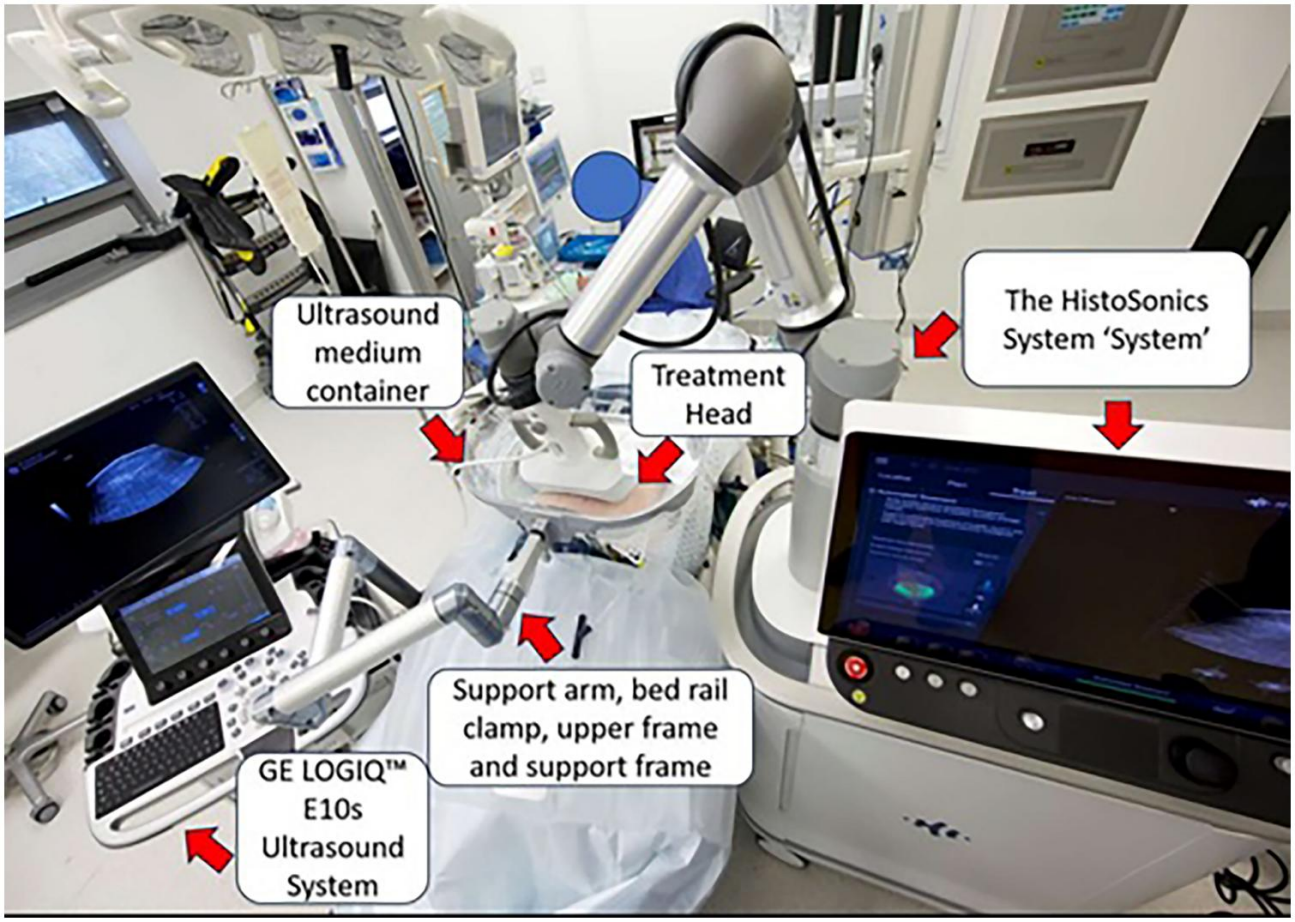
Image taken from Wah et al.^12^ Overhead view of the HistoSonics system components during histotripsy for treatment of kidney cancer from the CAIN trial. The GE LOGIQ™ E10s Ultrasound System and probe are integrated for tumour targeting, planning, and monitoring treatment. The treatment head contains both the histotripsy therapy transducer and an integrated ultrasound imaging probe. A multi-joint articulating support arm attaches to the bed rail, holding a reservoir that contains the ultrasound medium (degassed water) in position above the patient. A single-use patient membrane is inserted into the reservoir frame, creating a container for the ultrasound medium that conforms to the patient’s body, ensuring acoustic coupling between the treatment head and the patient.
